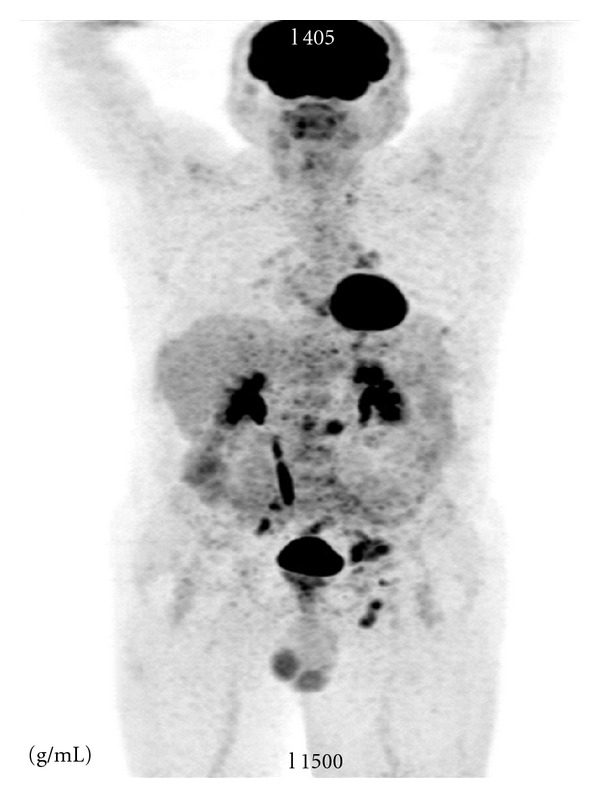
PET CT showed pathological increased FDG involvement of the left supraclavicular (suvmax: 4.5), aorticopulmonary (suvmax: 3.6), paratracheal (suvmax: 4.1), left tracheobronchial (suvmax: 5), left hilar (suvmax: 4.95), superior phrenic (suvmax: 5.1), paraaortic-aortocaval (suvmax: 8), common iliac (suvmax: 7.7), left external iliac (suvmax: 9), and left inguinale (suvmax: 7.15) lymph nodes.
